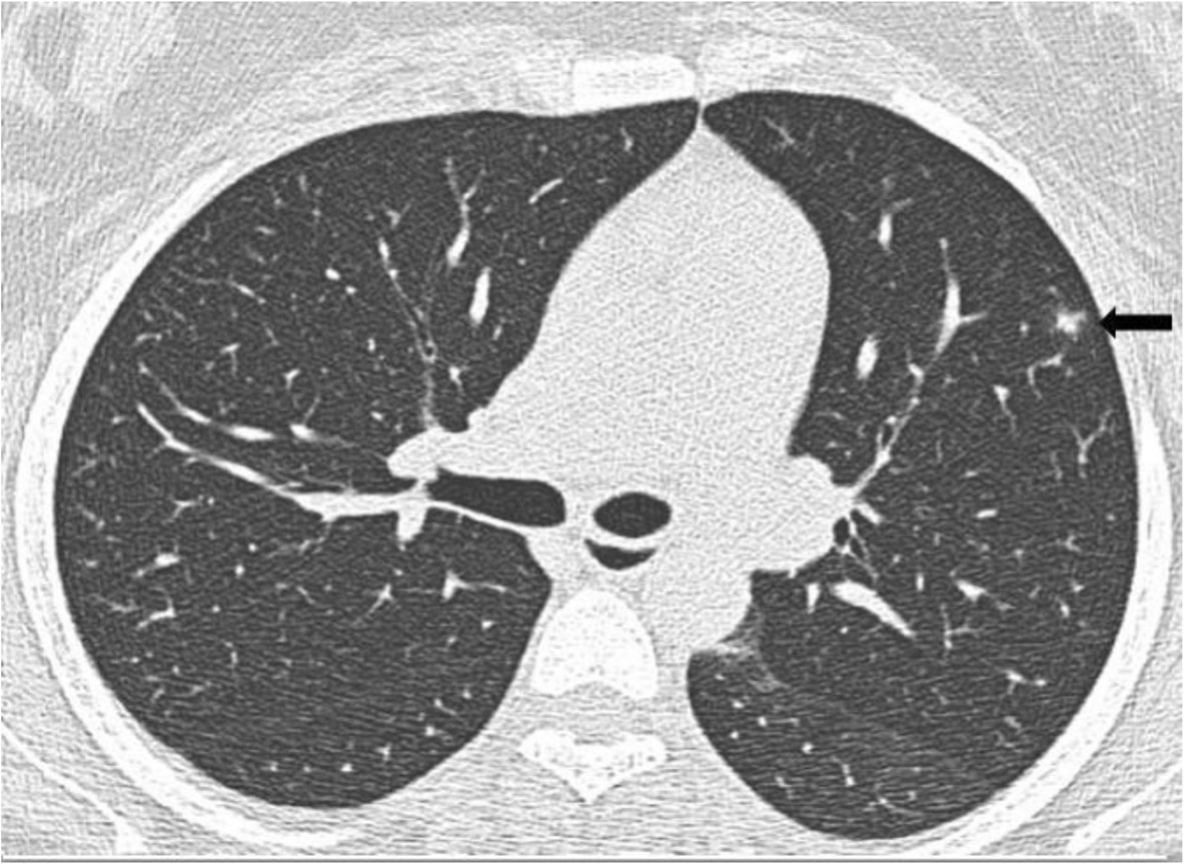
GGO nodule. Axial CT thorax of a 35-year-old COVID pneumonia patient showing small solitary GGO nodule in the left upper lobe as indicated by the arrow
